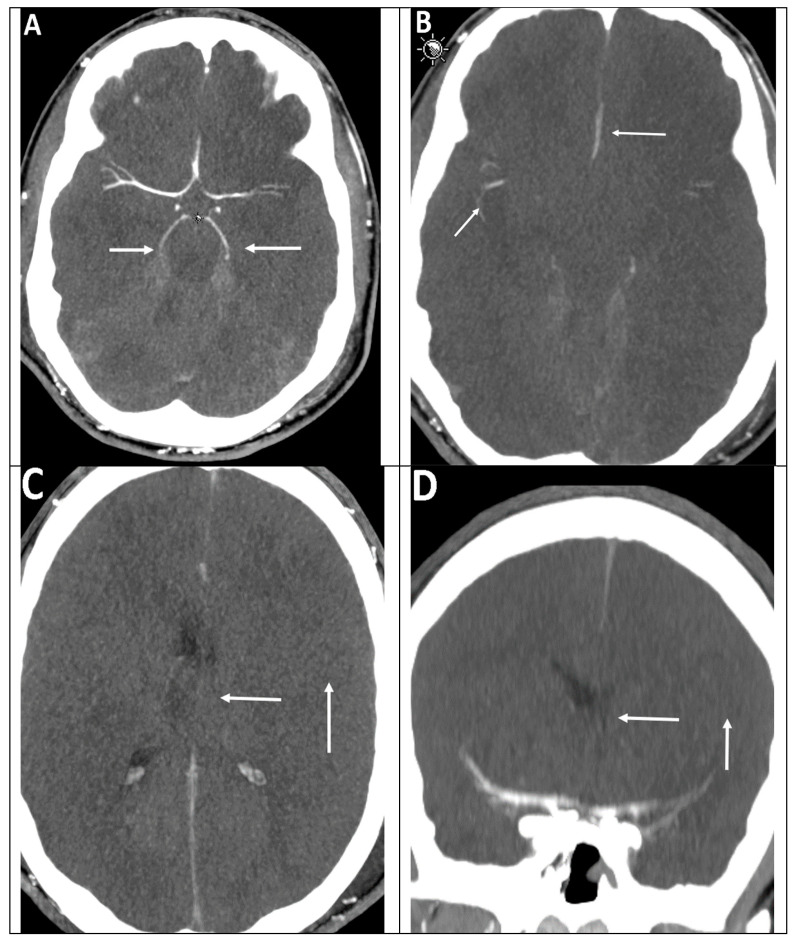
(A) Stasis filling in the proximal segments of ACA (A1, A2), MCA (M1, M2), and PCA (P1, P2, arrows). (B) Stasis filling also of the M3 segments of the MCA and the A3 segments of the ACA (arrows). The criteria of cerebral perfusion arrest are not fulfilled according to 7-point and 10-point CTA score. Axial (C) and coronal (D) CTA images demonstrating the lack of contrast filling of the M4 segments of the MCA and the ICV (arrows) consistent with the diagnosis of cerebral circulatory arrest according to the 4-point scale.
